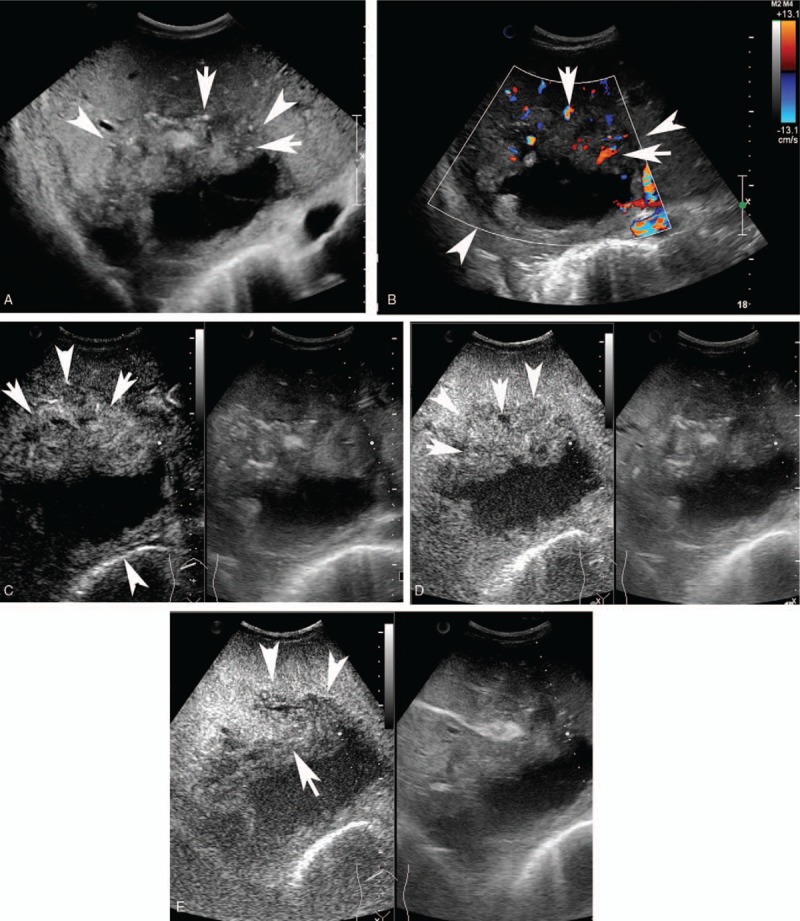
A 29-year-old man with hepatic AE. A: One typical AE lesion (arrow head) with dotted calcifications (arrow) was found in the liver. B: Abundant punctate and linear blood flow signals (arrow) were detected using color Doppler mod in the lesion (arrow head). C: CEUS showed irregular hyper-enhanced (arrow) in the arterial phase in the nodule (arrow head). D and E: In the portal phase and late phase, contrast agent wash-out heterogeneously (arrow) and the un-enhanced area remained. AE = alveolar echinococcosis, CEUS = contrast enhanced ultrasound.
